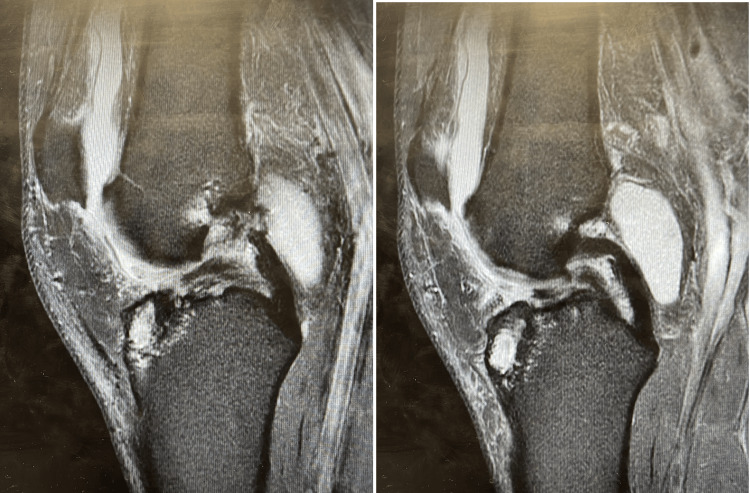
T2-weighted sagittal magnetic resonance imaging (MRI) scans of a 46-year-old male patient showing torn anterior cruciate ligament (ACL).
